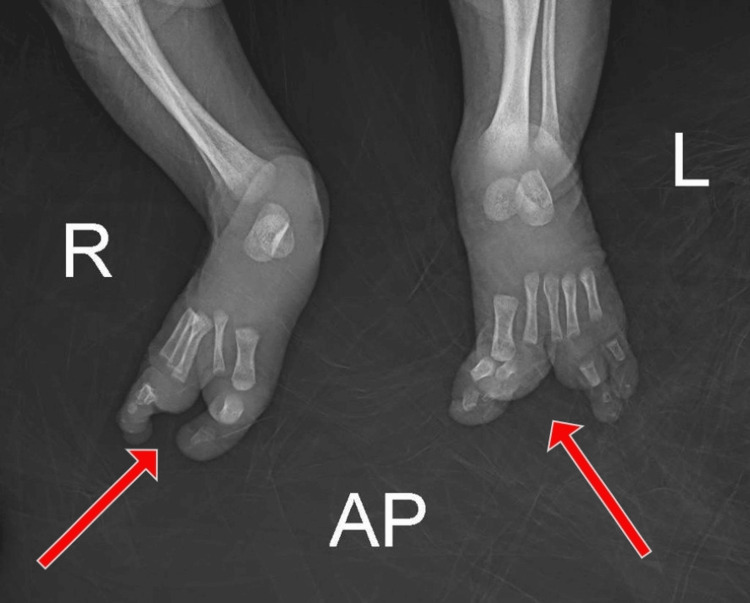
Plain radiograph of both feet showing the left foot missing the phalanges of the second and third toes, and the right foot missing the phalanges of the second toe
